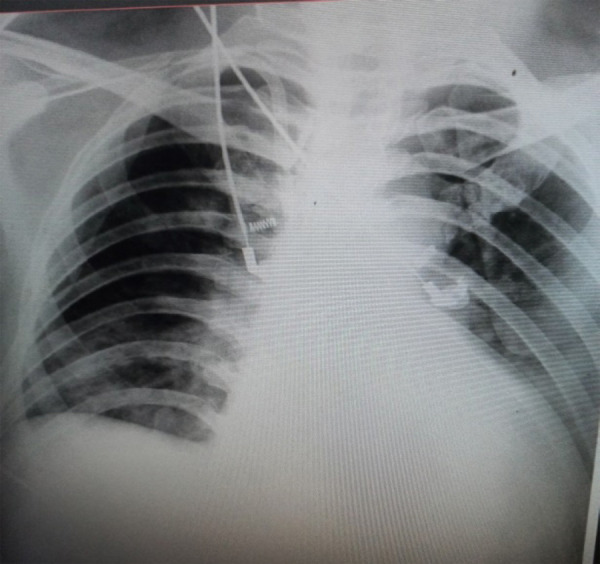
Chest X-Ray (Antero-posterior view) taken in the intensive care unit on the second day of admission.
